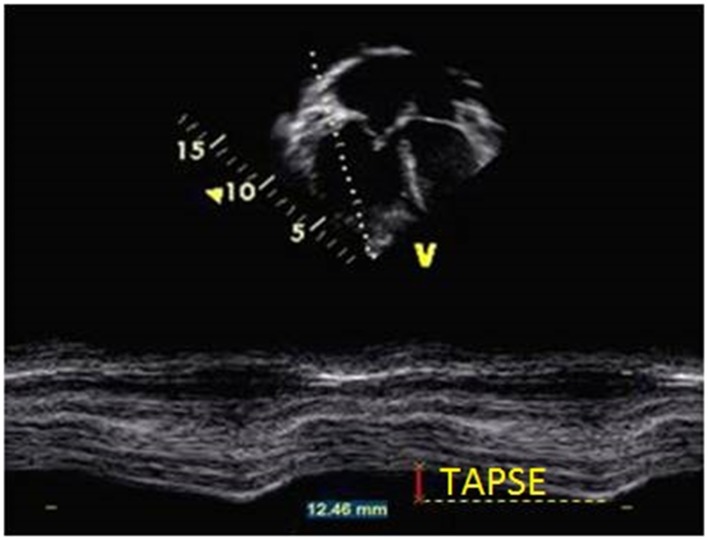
Measurement of tricuspid annular plane systolic excursion (TAPSE) from M-mode in the apical four-chamber view at the level of the tricuspid annulus in a patient with pulmonary hypertension.
